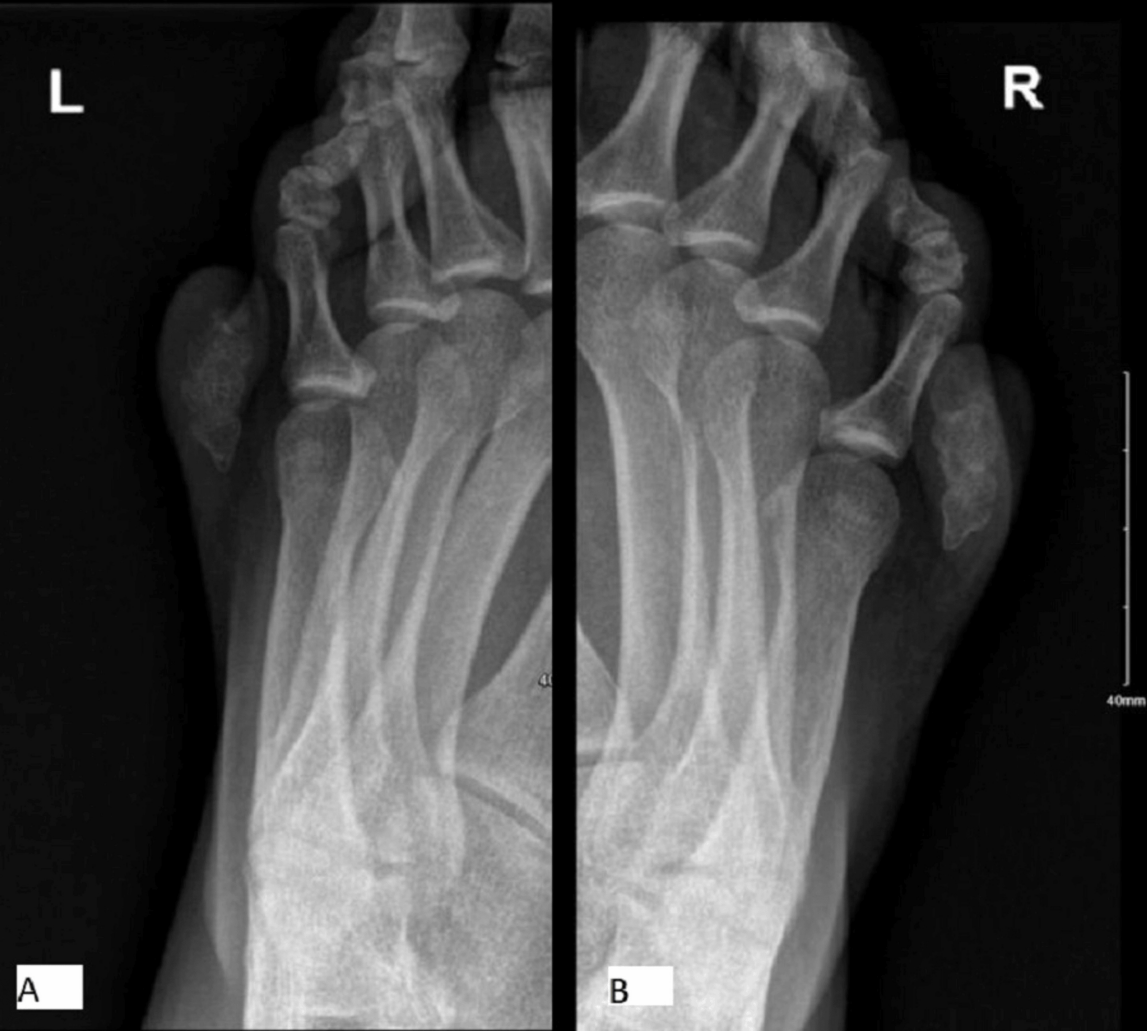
Bilateral foot X-rays showing ossification of the extra digits. (A) Left foot. (B) Right foot.
